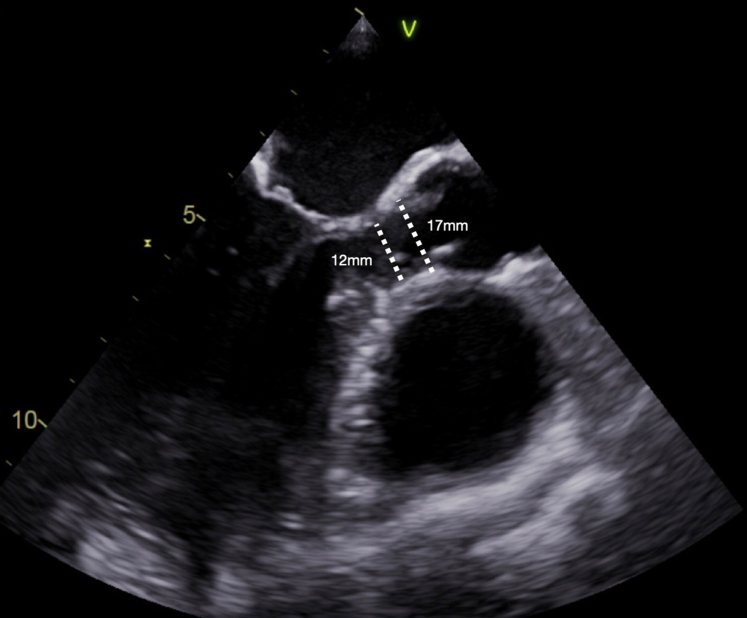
Transesophageal echocardiography, preoperative long-axis view showing the aortic valve and left ventricular outflow tract (in systole) and its diameters.
